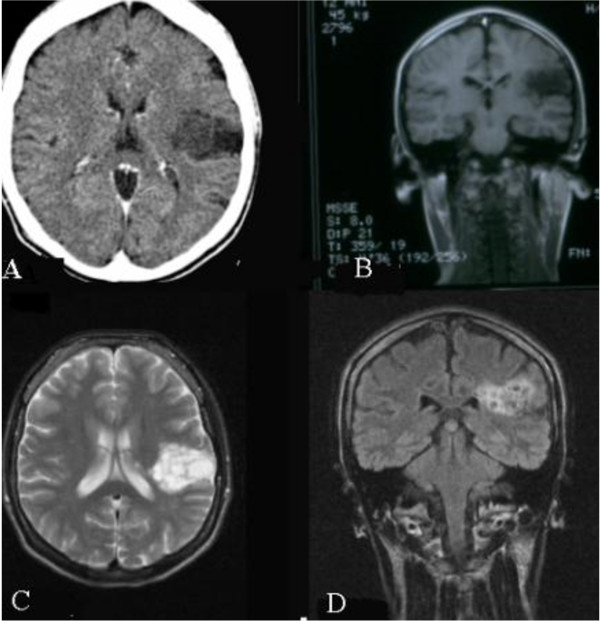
Imaging results. (A) First CT scan show a left temporoparietal diffuse hypodense area, quite inhomogeneous without mass effect. (B- D) MRI performed 13 years after seizure onset revealed a multicystic cortico-subcortical parietal lesion, without edema, mass effect, and enhancement.
